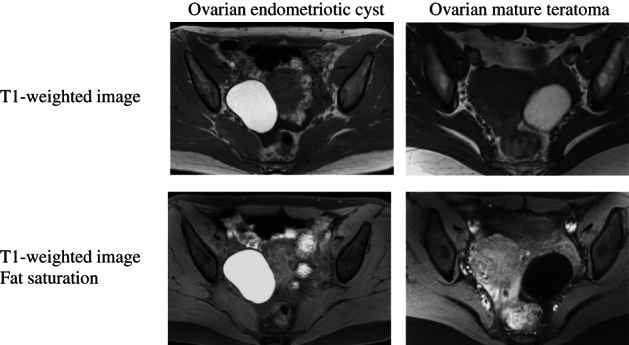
MRI findings. Ovarian mature teratomas are visualized with low signal intensity due to suppression of fat signals (lower right), whereas ovarian endometriotic cysts are not suppressed and continue to be visualized with high signal intensity (lower left).
